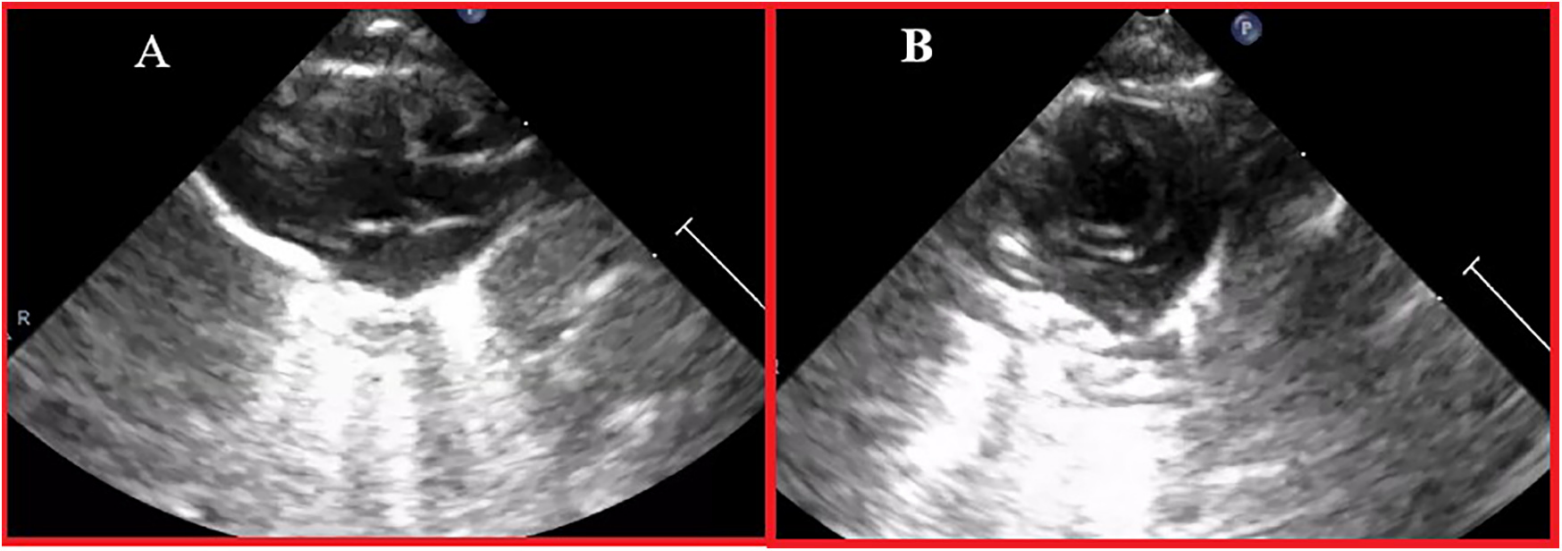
Echocardiographic image example. (A) Parasternal long axis view; (B) Parasternal short axis view.
